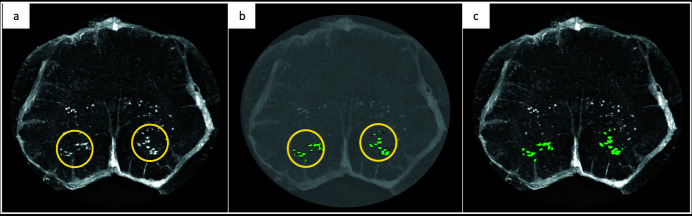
X-ray phase contrast tomography images of the lumbar region of the spinal cord. Example of the MN 3D segmentation process. (*a*) A ROI was selected in the ventral horn of the spinal cord where the MNs are anatomically concentrated. (*b*) An intensity threshold segmentation was performed to isolate MNs. (*c*) Superimposed image of (*a*) and (*b*) to show the output of the segmentation process.
